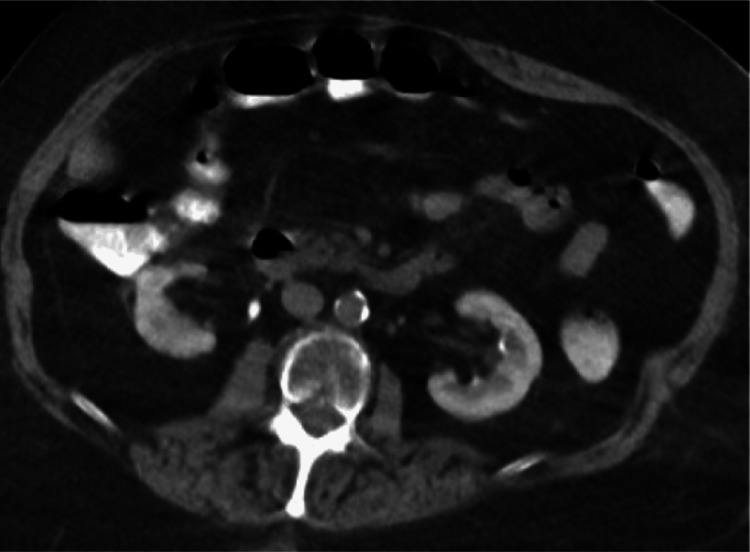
CT scan to rule out malignancy, no abnormalities noted in the abdominal region other than severe cortical thinning of the bilateral kidneys. No pancreatic abnormalities.
